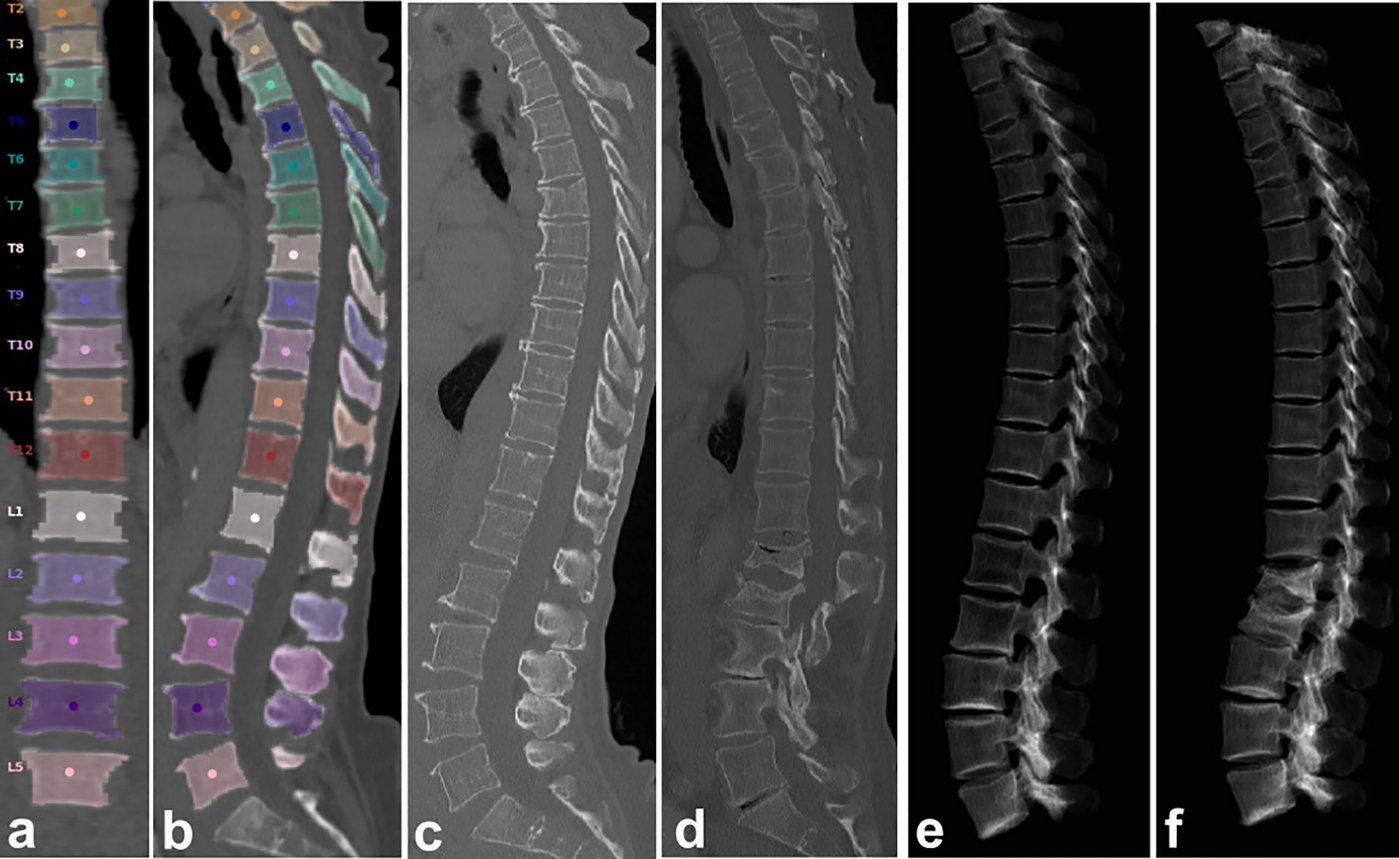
Automated spine labeling and segmentation in coronal (A) and sagittal (B) view of a baseline CT of a 79-year-old female with trabecular vBMD (L1-L4) = 48.9 mg/cm³. (C, E) CT and virtual radiograph at baseline. (D, F) CT and virtual radiograph at follow-up after 8 months showing incident vertebral fractures of L1 and L2.
